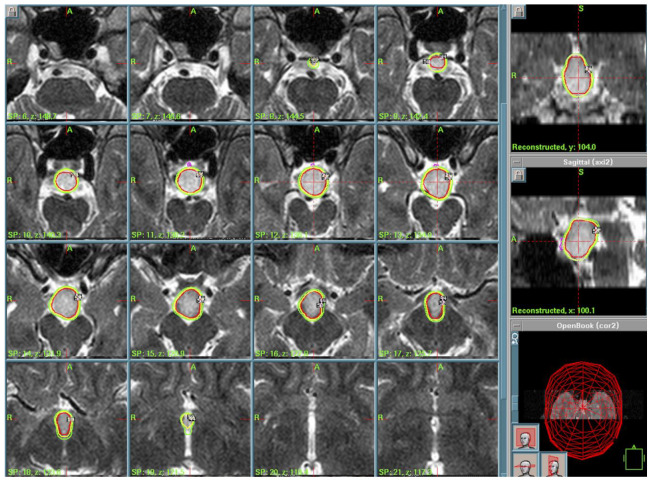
Case 3 treatment planning. Axial T2-weighted MRI view. In red is the contouring of the lesion; in green and yellow is the 50% isodose line, avoiding dose exposure for the brainstem <15 Gy.
